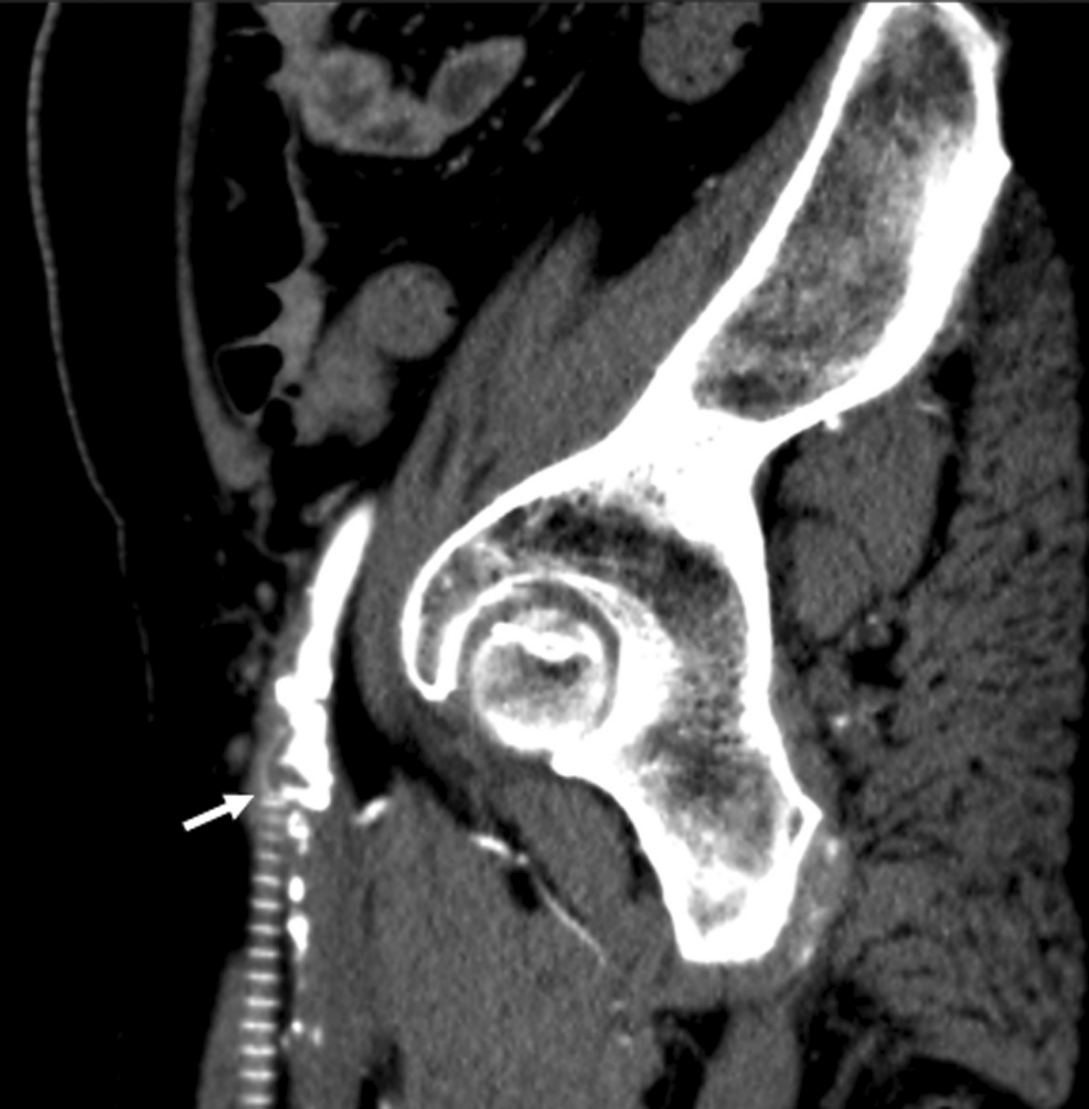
Preoperative computed tomography reveals projection of artificial ePTFE graft vessel thrombosis into the common femoral artery lumen (arrow). ePTFE, expanded polytetrafluoroethylene.
